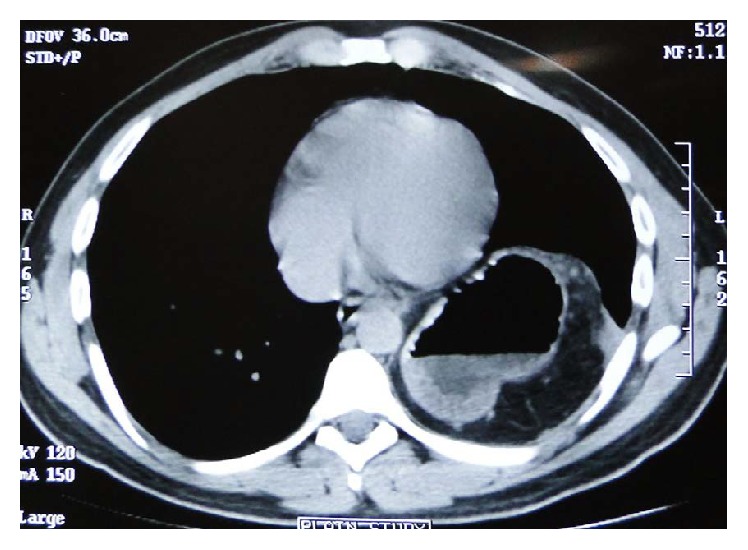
CT scan showing stomach herniation in left Bochdalek hernia.
